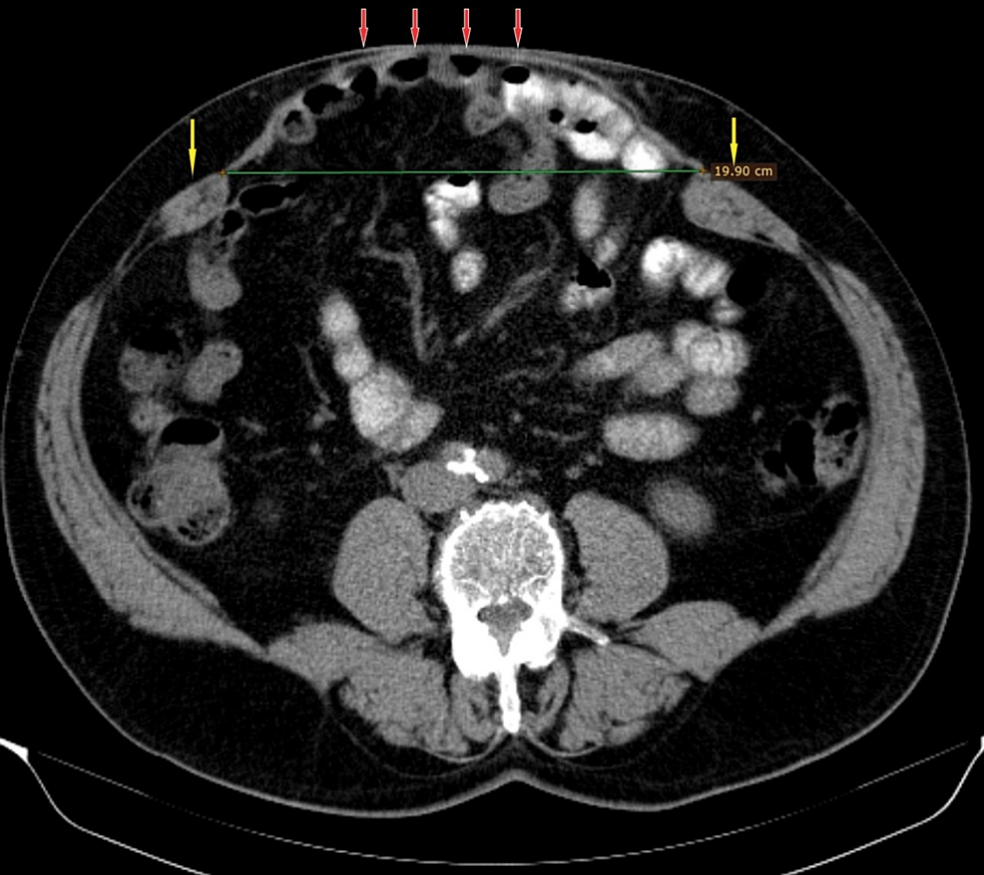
Preoperative abdomen and pelvis CT scan The image shows a giant incisional ventral hernia with a midline defect, maximum 19.9 cm in width, wide displacement and atrophy of the rectus abdominis muscle (yellow arrows), and herniated bowel loops adherent to the skin (red arrows) CT: computed tomography
